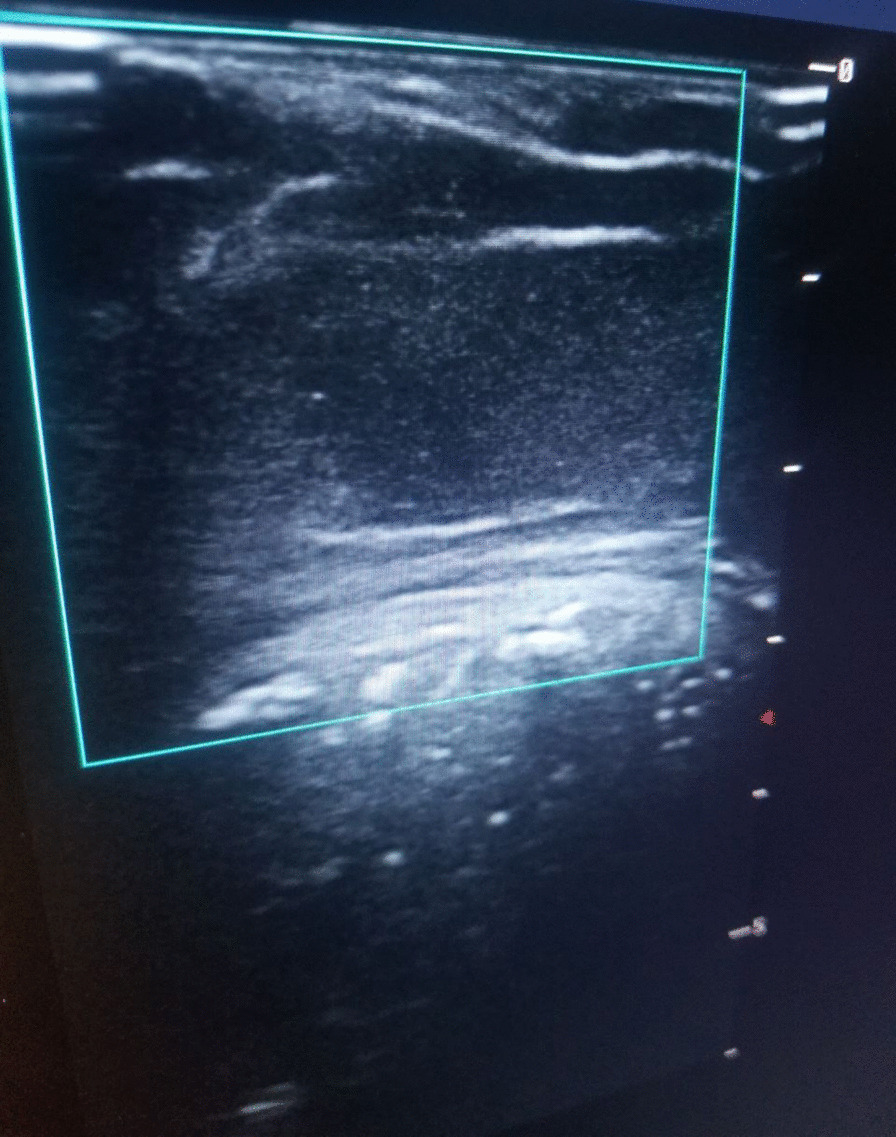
Neck ultrasound of thyroid tuberculosis revealing abscess collections on the left lobe of thyroid on the background of multinodular goiter
